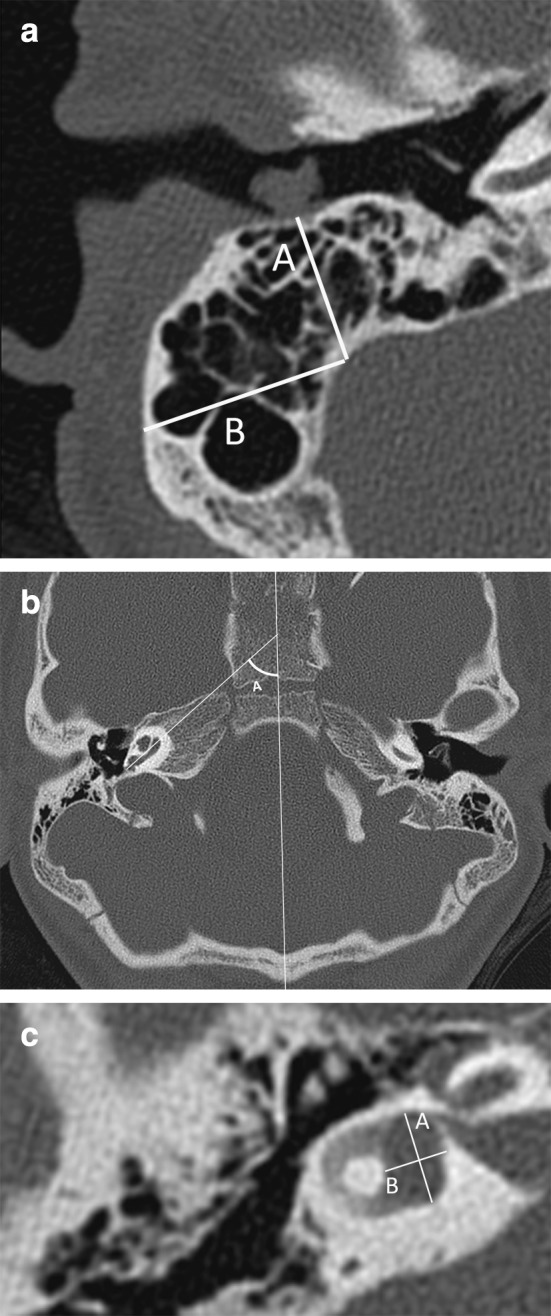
Measurements in axial CT images. a Mastoid size *A* anterior-posterior (AP) size, measured in the middle of the external meatus (cranial/caudal) as the minimal distance from the external meatus to sigmoid sinus *B* lateral-medial (LM) size, distance between outer cortex and sigmoid sinus, measured perpendicular to A. b Angle cochlear basal turn. c Vestibulum size *A* longitudinal extension, *B* transversal diameter (right ear)
